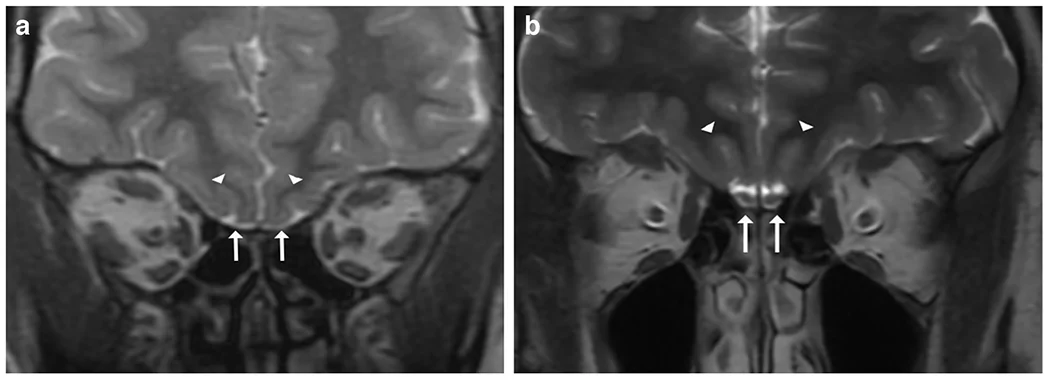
Fourteen-year-old male with anosmia and hypogonadotropic hypogonadism (Kallman syndrome). a Coronal T2-weighted sequence through the bifrontal region shows absence of the bilateral olfactory tracts in their expected locations (*arrows*). Shallow olfactory sulci are also noted (*arrowheads*). b For comparison, coronal T2-weighted image through the bifrontal region of a 15-year-old boy with the ability to smell shows a normal cross-sectional appearance of the bilateral parallel olfactory tracts (*arrows*) and deep olfactory sulci (*arrowheads*)
